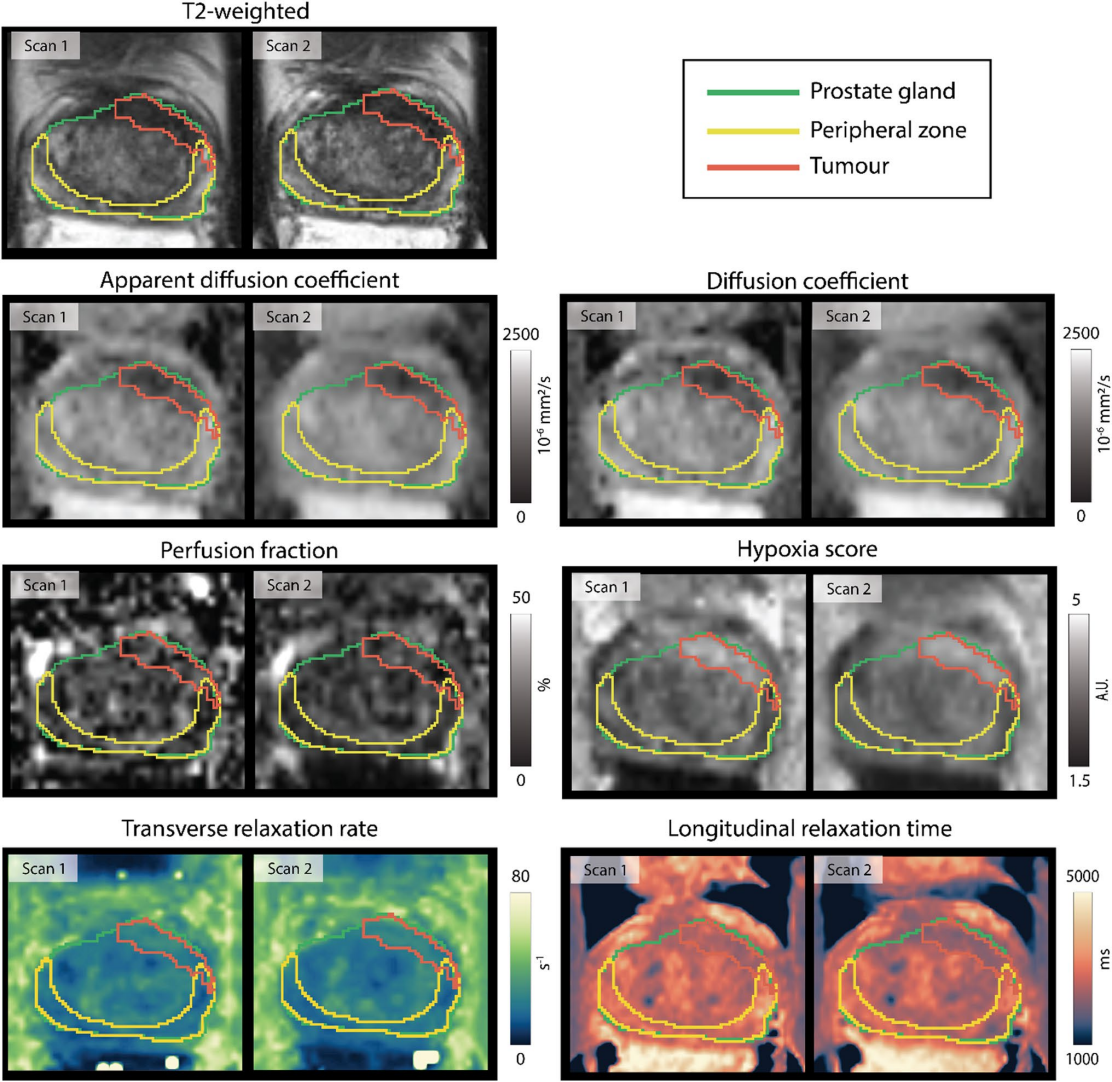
Example of the registered test–retest axial T2-weighted images, apparent diffusion coefficient, diffusion coefficient, perfusion fraction, hypoxia score, transverse relaxation rate, and longitudinal relaxation time maps of a patient. All images were registered to the sagittal T2-weighted image of the first scan. The prostate gland, peripheral zone, and tumor region of interests, delineated on the sagittal T2-weighted image of the first scan, were propagated to each qMRI parameter map and axial T2-weighted image
